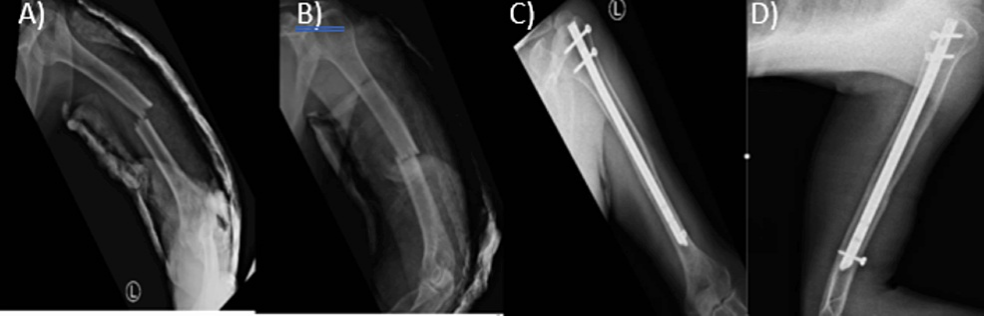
Anteroposterior (AP) and lateral radiographs of the left humerus shaft fracture fixed with an antegrade intramedullary nail (AIMN). (A) Preoperative anteroposterior (AP) view, (B) preoperative lateral view, (C) postoperative antegrade intramedullary nail (AIMN) fixation AP view, (D) postoperative AIMN fixation lateral view
